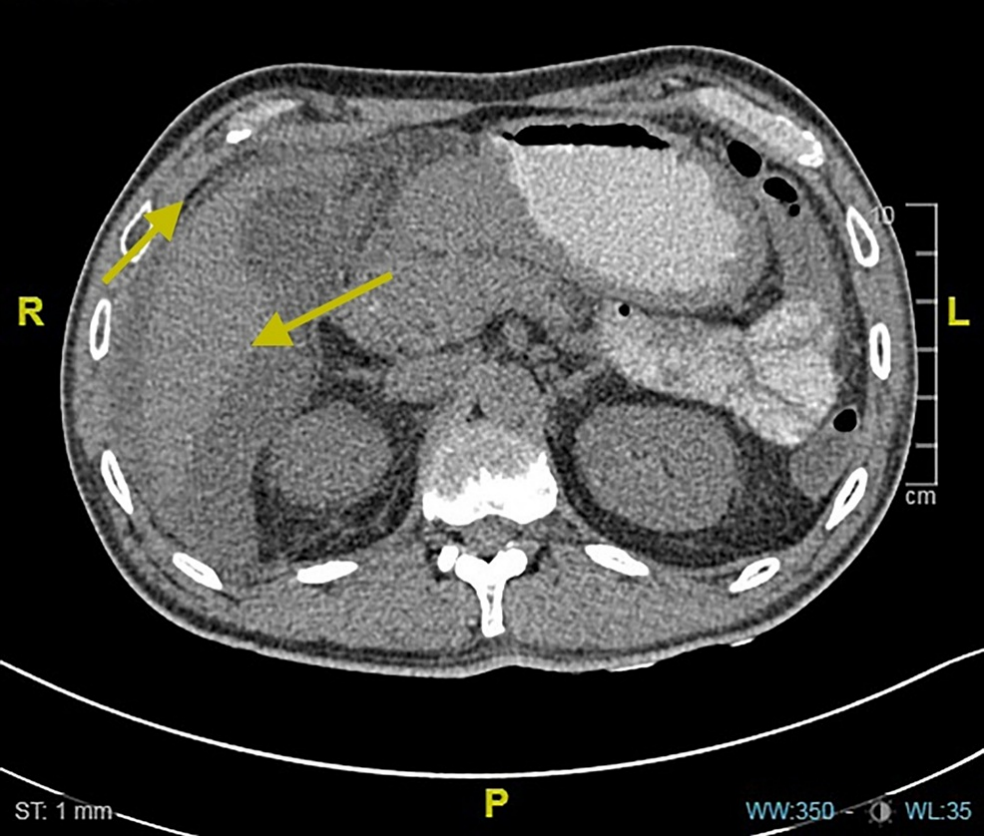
Abdominal CT-scan shows pneumoperitoneum secondary to perforated viscus (right yellow arrow) and peri-hepatic free fluid with sub-capsular liver collection (left yellow arrow).
